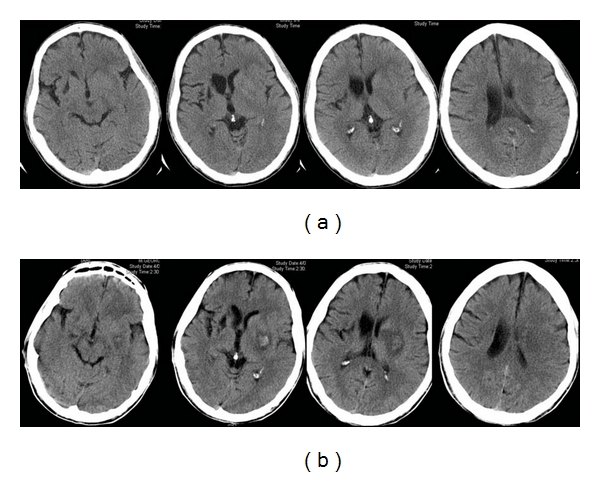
78-year-old man presented with right hemiparesis and dysphasia. (a) Axial unenhanced CT images 5 hours after stroke show an area of low attenuation in the left lentiform nucleus. (b) Repeated axial unenhanced CT images 3 days later show a parenchymal hematoma within the area of infarct. Stage HI d2 [1]. Even if the initial CT images are not available, the topography of the stroke is suspicious for hemorrhagic infarct. The centre of the hematoma is in the striatocapsular region with the surrounding hypodensity extending superiorly, following the topography of the middle cerebral artery.
